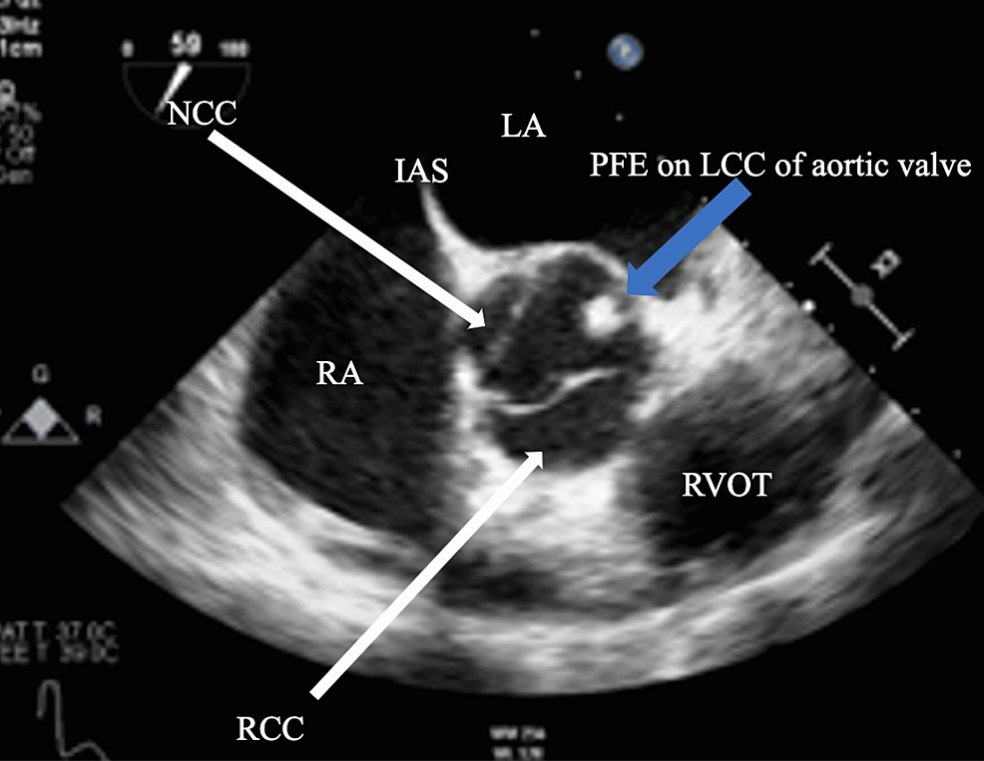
Midesophageal view of the TEE showing the papillary fibroelastoma on the left coronary cusp of the aortic valve (blue arrow) RA: right atrium, LA: left atrium, RVOT: right ventricular outflow tract, IAS: inter atrial septum, RCC: right coronary cusp, LCC: left coronary cusp, NCC: non-coronary cusp, PFE: papillary fibroelastoma, TEE: transesophageal echocardiogram
